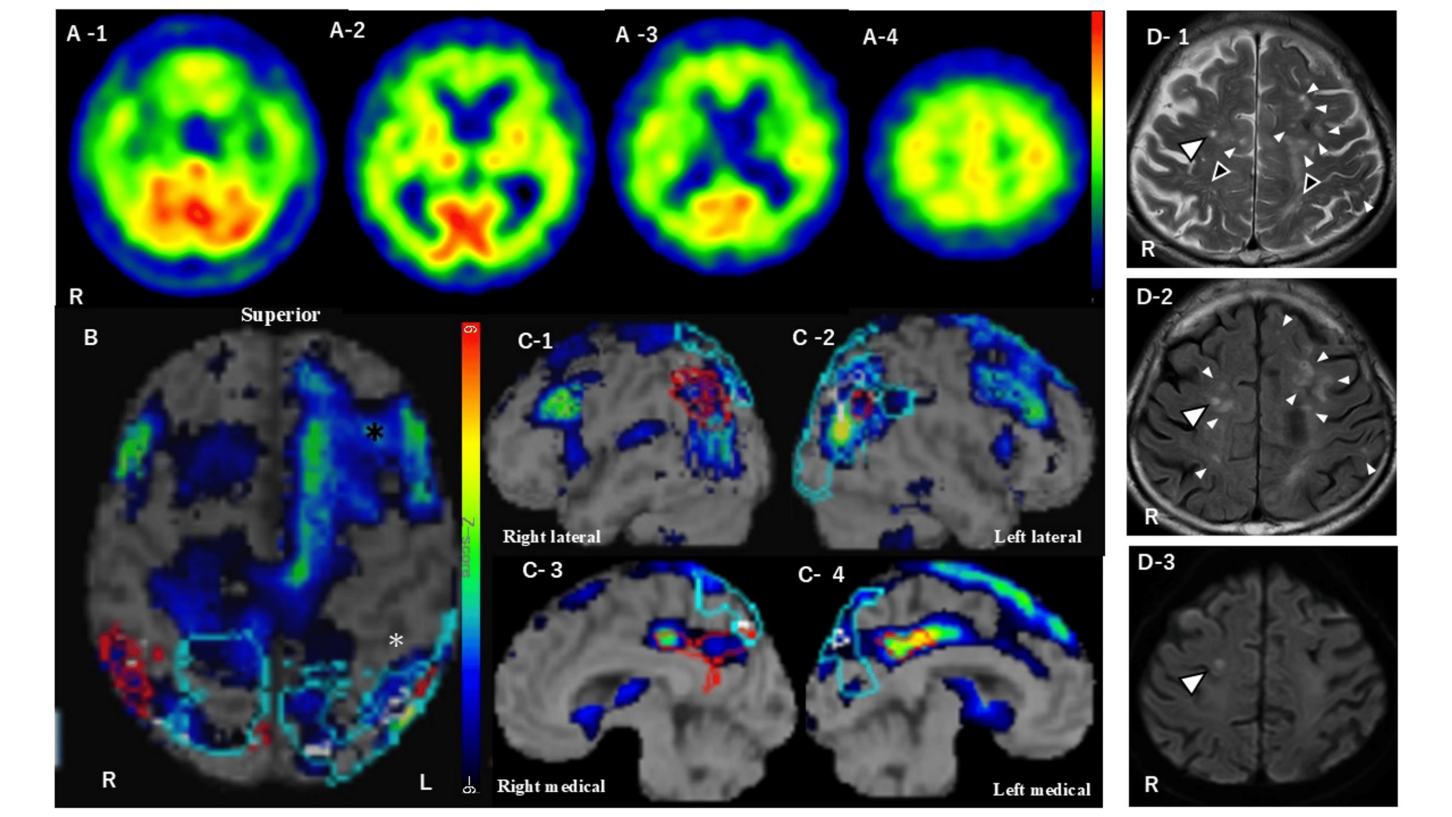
Cerebral blood perfusion SPECT and MRI findings 10 months after initial visit A-1 to A-4: Cerebral perfusion SPECT revealed a generalized reduction in cortical blood flow. In contrast, perfusion in the basal ganglia and thalamus was relatively preserved bilaterally. B, C-1 to C-4: The Z-score maps generated by the eZIS showed marked hypoperfusion in the left frontal lobe (black asterisk), with additional reductions in the right frontal lobe, bilateral parietal lobes, and the bilateral posterior cingulate gyrus. Notably, no hypoperfusion was observed in the left postcentral sulcus or adjacent regions (white asterisk). D-1, D-2: The T2WI (D-1) and FLAIR (D-2) axial MRI images. The CSO-PVS are visible on T2WI (black arrowheads). The WMH-MS are evident in both images, predominantly in the frontal lobes with left-sided dominance (white arrowheads and large white arrowhead). D-3: One hyperintense spot was detected among WMH-MS in the right frontal lobe on DWI (large arrowhead) SPECT: Single-photon emission computed tomography; eZIS: Easy Z-score imaging system; T2WI:T2-weighted imaging; FLAIR: Fluid-attenuated inversion recovery; DWI: Diffusion-weighted imaging; VPS-CSO: Visible perivascular spaces in the centrum semiovale; WMH-MS: White matter hyperintensities in a multispot pattern
